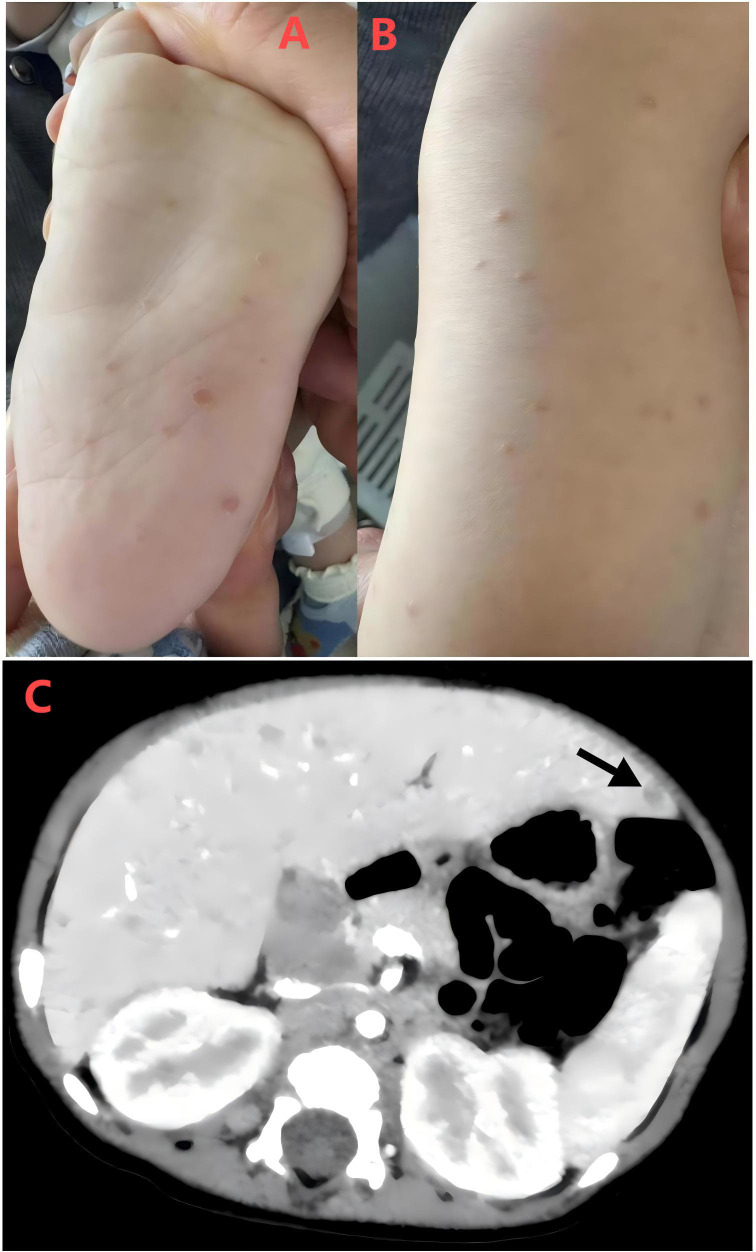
(A) Multiple reddish, round-to-oval macules on the infant’s sole, with some lesions exhibiting epidermal disruption on the surface. (B) Multiple well-circumscribed, erythematous, discrete papules distributed over the infant’s lower limbs. (C) Contrast-enhanced abdominal CT demonstrates diffuse cystic low-attenuation lesions within the liver (arrows). The largest lesion (~ 6 × 5 cm) shows ill-defined margins with mild peripheral enhancement, findings suggestive of an infectious process.
